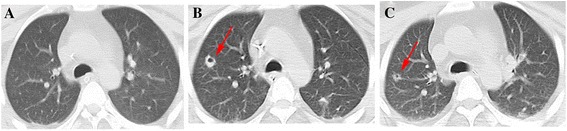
Computed tomography images of lung. a Computed tomography scan on day 4 showing no abnormality. b Computed tomography scan on day 23 showing emerging nodules with cavity. c Computed tomography scan on day 38 showing narrowing nodules. *Red arrows*, cavity
